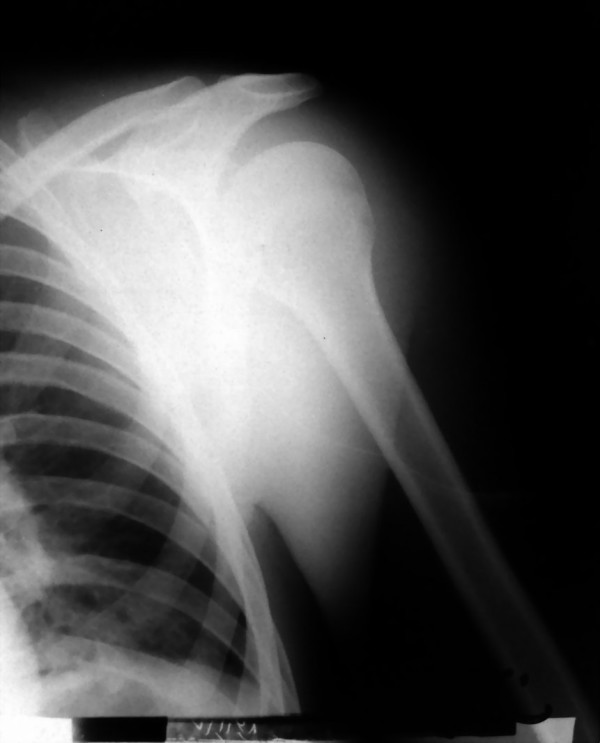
Post-operative x-ray shows reduction.
